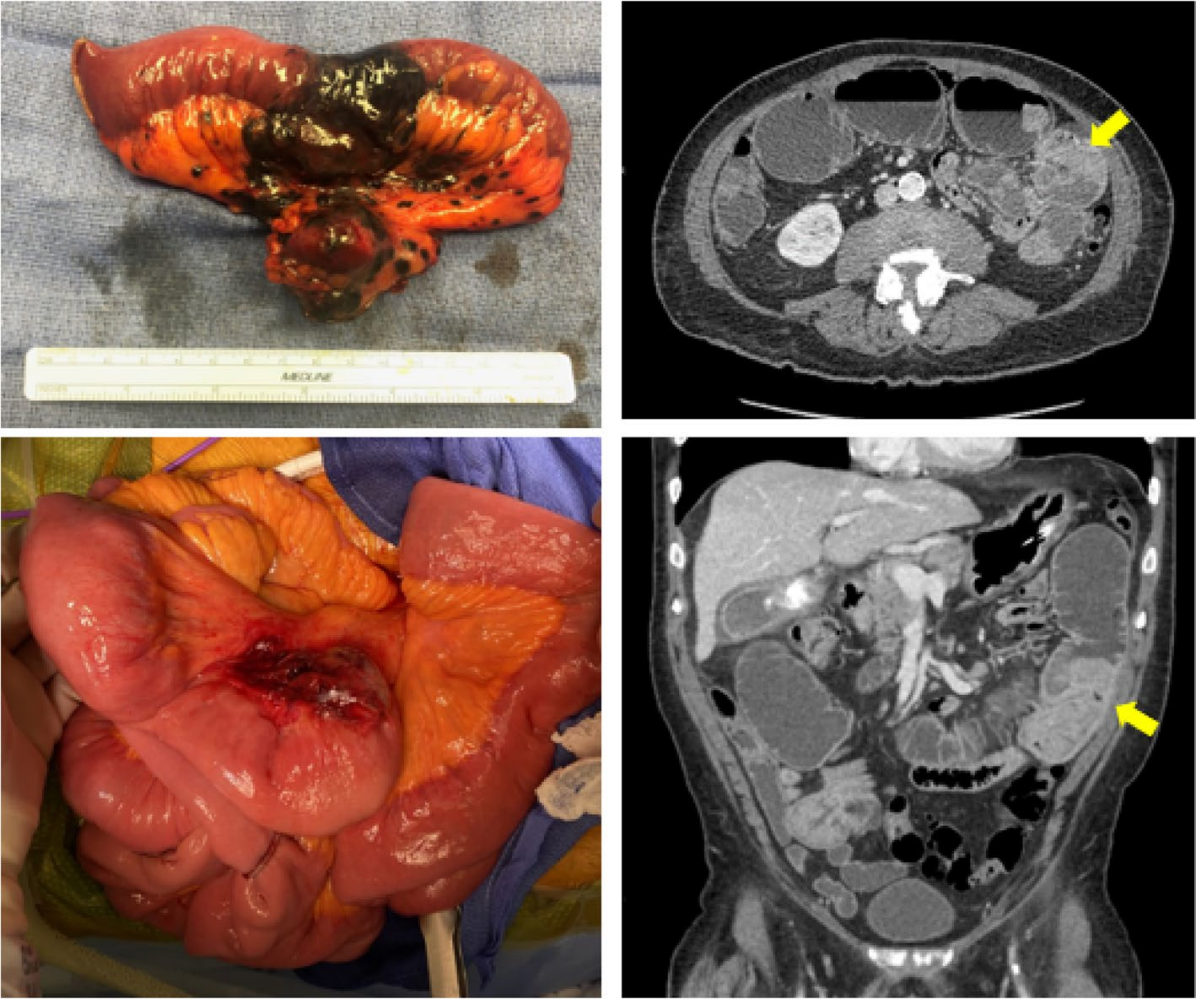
Left panels: intraoperative examples of metastatic melanoma lesions to small bowel. The upper left panel demonstrating melanosis, while the lower left depicts mesenteric invasion. Right panels: axial and coronal CT images of intraluminal metastatic melanoma causing obstruction
